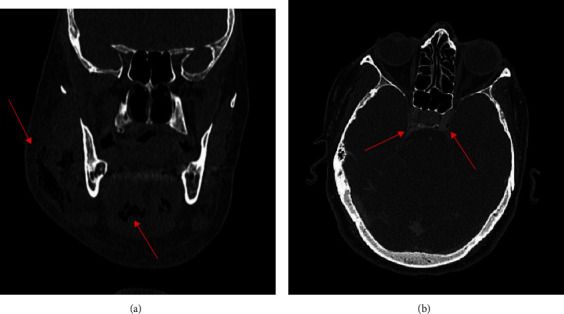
(a, b) Initial computed tomography (CT) of the head: frontal plane (a); transverse plane (b). Presence of gas bubbles in both cavernous sinuses, the right half of the face and buccal floor (red arrows) (13/02/2021).
